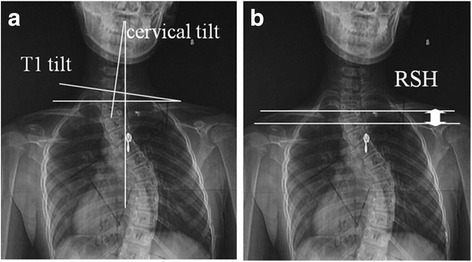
a and b Cervical tilt: the angle is formed by the intersection of the *vertical line* and the longitudinal axis of the cervical spine (a); T1 tilt: angle between the *line* along the superior endplate of T1 and a perpendicular to the *horizontal line* (a); Radiographic shoulder height (RSH): the difference in the soft tissue shadow directly superior to the acromioclavicular joint (the value is defined as positive when left shoulder is up and negative when right shoulder is up) (b)
